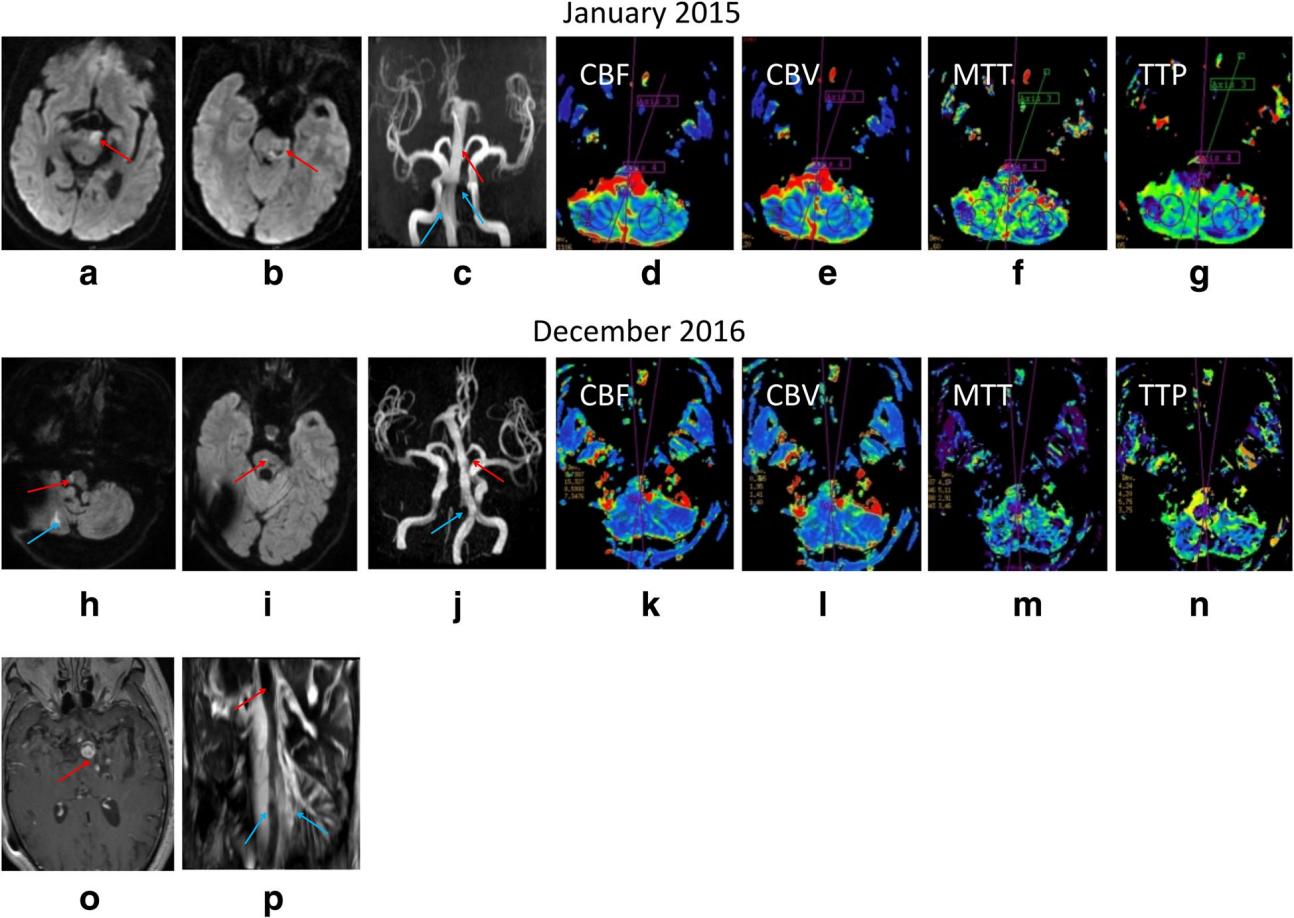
Follow-up multi-modal MRI scans. (a) DWI showing acute ischemic infarction in the left mesencephalon-pontine junction (red arrow). (b) DWI showing old infarction with reactive gliosis (red arrow). (c) TOF-MRA demonstrating an abnormal BA caliber (red arrow), bifurcation at the third ventricle floor, and bilateral vertebral arteries are present with enlarged diameters (blue arrow). (d-g) PWI showing decreased cerebral blood volume (CBV) and cerebral blood flow (CBF) of the medulla oblongata, pons, and cerebellum, and elongated MTT and TTP of the right cerebellum. (h) DWI showing acute ischemic infarction in the right medulla oblongata (red arrow) and cerebellum (blue arrow). (i) DWI showing acute ischemic infarction in the right pons with Wallerian degeneration (red arrow). (j) TOF-MRA showing an enlarged diameter and increased length of the BA (red arrow), bifurcation at the level of the bilateral ventricles, and non-observable right vertebral artery (blue arrow). (k-n) PWI shows decreased CBV and CBF of the bilateral medulla oblongata, and cerebellum, and elongated TTP of the right medulla oblongata. (o) High-resolution MRI showing signs of a double lumen of the BA (red arrow). (p) Basi-parallel anatomic scanning showing a larger diameter of the BA than that seen with TOF-MRA (red arrow) and an observable right vertebral artery (blue arrow)
